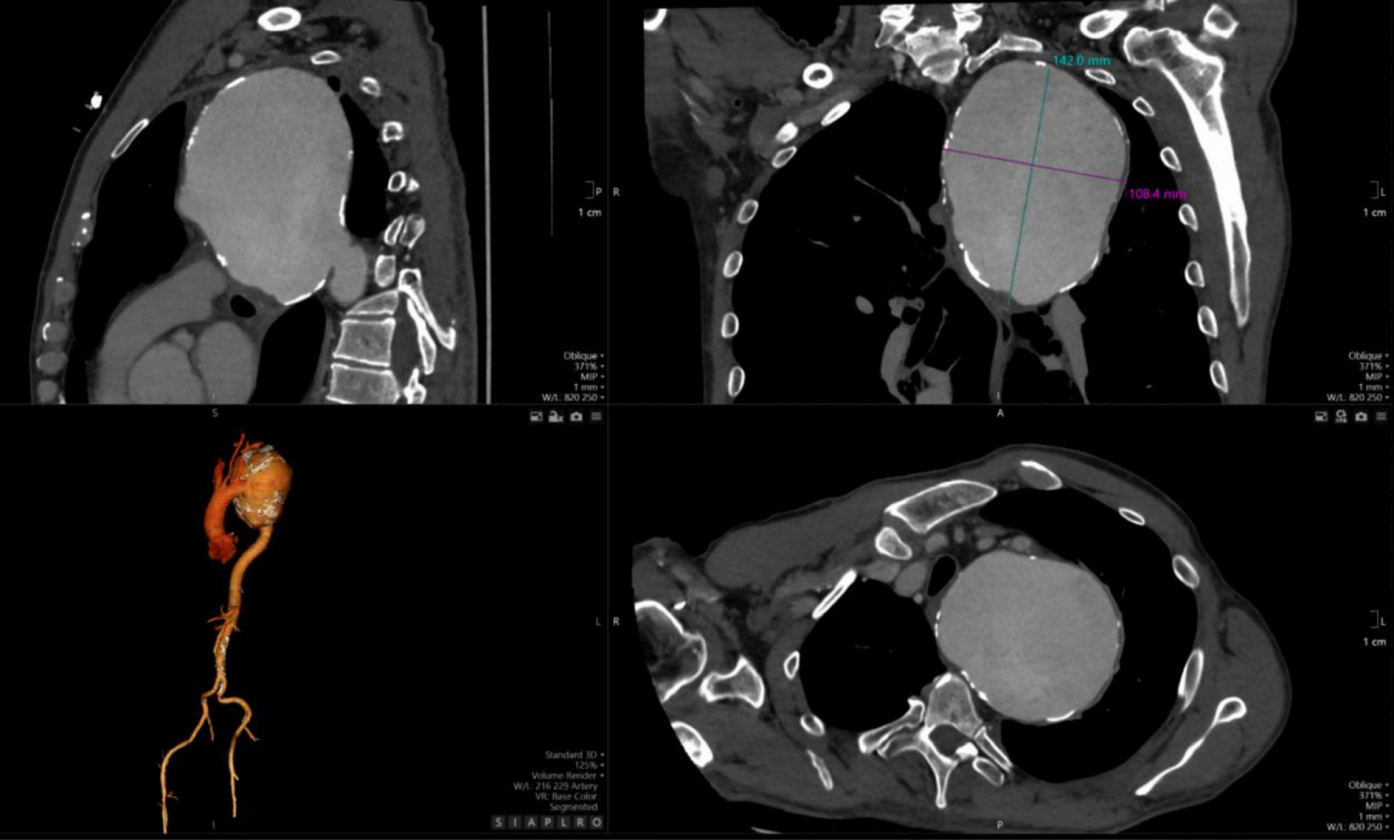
2-D CT, showing sagittal, oblique transverse section of aneurysmal thoracic aorta
